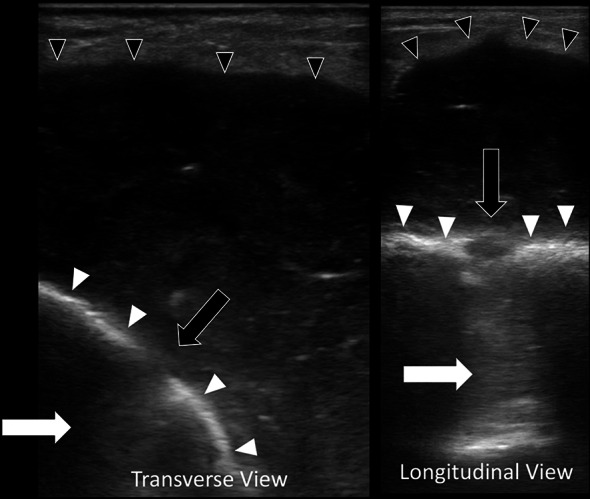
Point-of-care ultrasound demonstrating a heterogeneous hypoechoic fluid collection (black arrowheads) adjacent to the tibial cortex (white arrowheads). There is a defect in the cortex (black arrow) with fluid communicating into the medullary cavity (white arrow)
